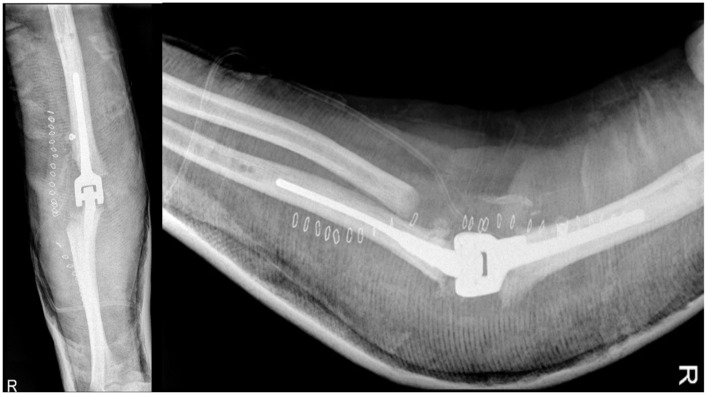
X-ray anteroposterior and lateral views of the right elbow after the placement of the total elbow arthroplasty (TEA) with the restoration of normal joint relationships.
